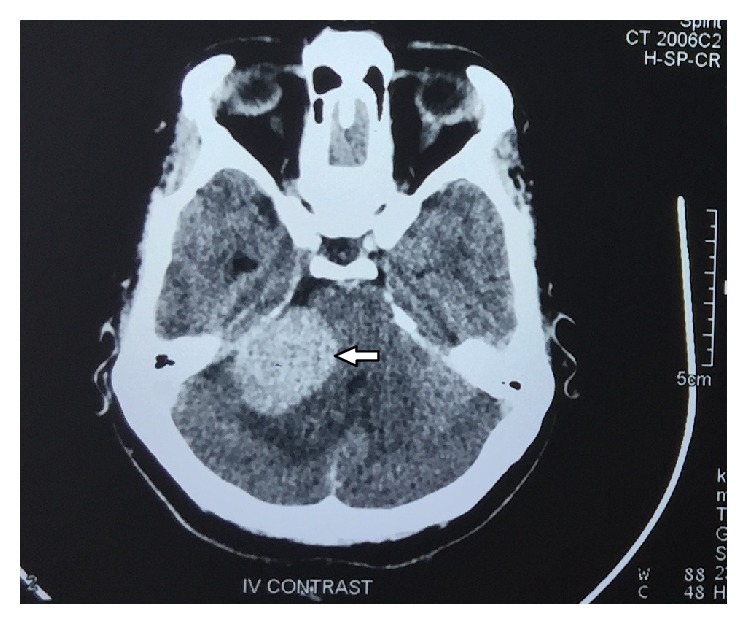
Contrast axial CT shows an ovoid homogeneous enhancing lesion (38 mm × 27 mm) involving right cerebellopontine cistern (white arrow).
